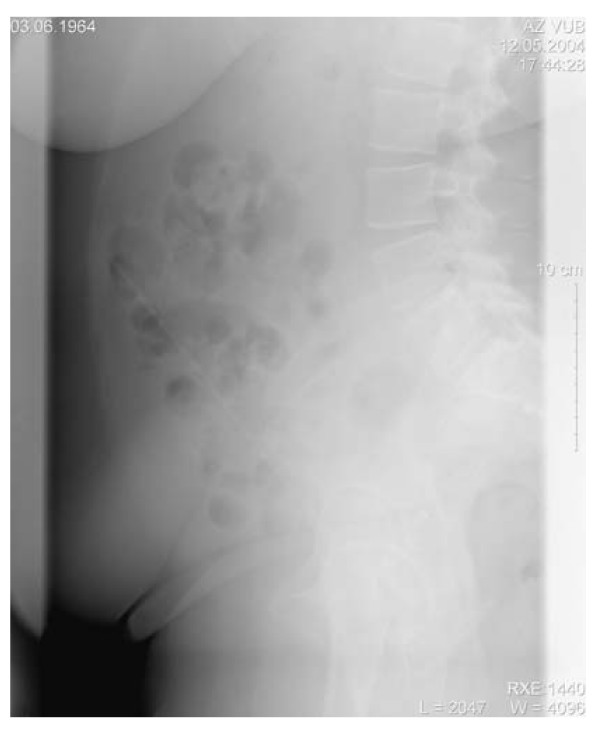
An abdominal X-ray confirmed the diagnosis of an intra abdominal foreign body.
